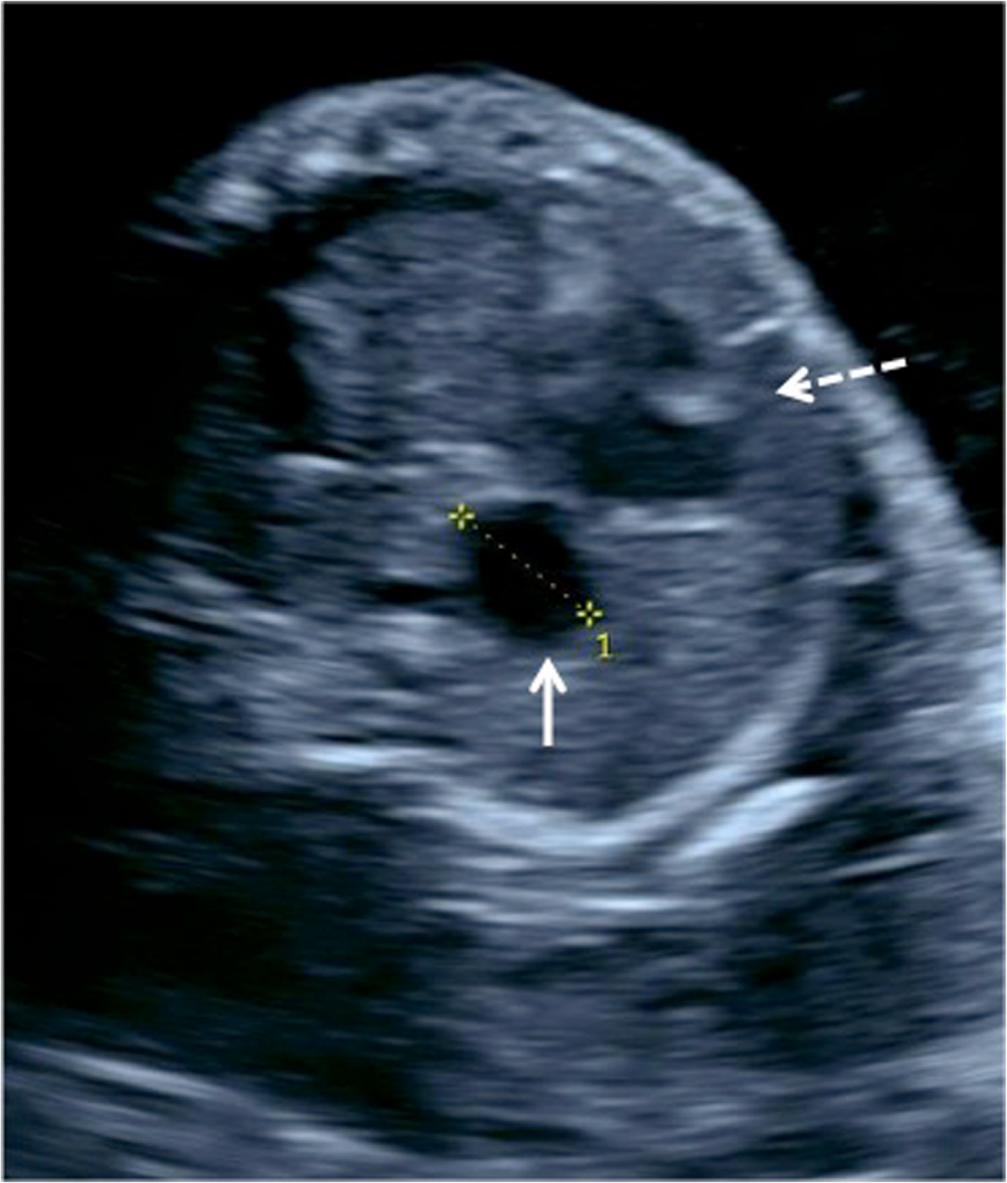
Transverse ultrasound view of the fetal chest showing the cystic structure (*white arrow*) posterior to the heart (*dashed arrow*)
